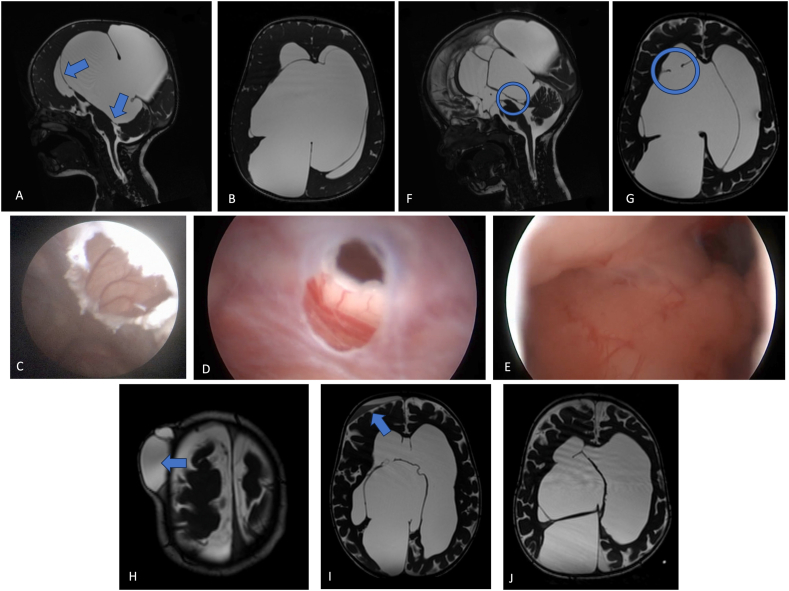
A: A sagittal CISS MRI of a 10-months old child who presented with macrocephaly, MRI showed a huge quadrigeminal arachnoid cyst compressing the whole ventricular system and nearby structures causing occlusive hydrocephalus, the blue arrows show the site of the planned fenestration to right lateral ventricle and 4th ventricle as aqueduct stenosis could not be excluded from preop. MRI. B: an axial CISS MRI showing the massive space occupying effect of the cyst exerted on the ventricular system. C: Intraoperative image showing the fenestration to right lateral ventricle. D: Intraoperative image showing the view inside the fenestrated 4th ventricle. E: Intraoperative image showing the fenestration through the roof of 4th ventricle and showing Foramen of Magendie. F: Postoperative CISS MRI showing the open aqueduct after release of pressure exerted from dorsally. G: An axial CISS MRI showing the performed fenestration to the right lateral ventricle. H: An axial CISS MRI showing the CSF collection subcutaneous at the site of the bur-hole. I: An axial CISS MRI showing the subdural hematoma. J: An axial CISS MRI 2 years after surgery resolution of CSF subcutaneous and subdural collections without new surgical interventions.
